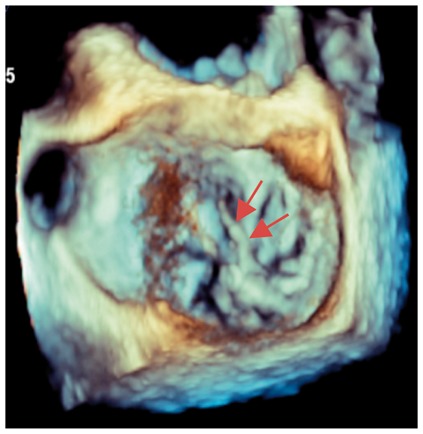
Colour Doppler	Colour Doppler	Colour Doppler	CMR en-face valve view
